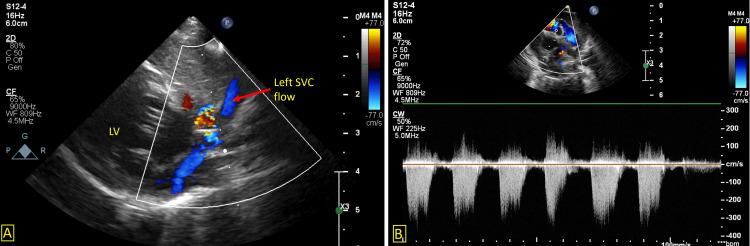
A: Color Doppler of a high parasternal view showing a left superior vena cava. B: Showing a maximum gradient of 50 mmHg on continuous wave Doppler of the right ventricular outflow tract. LV – Left ventricle, SVC – Superior Vena Cava
